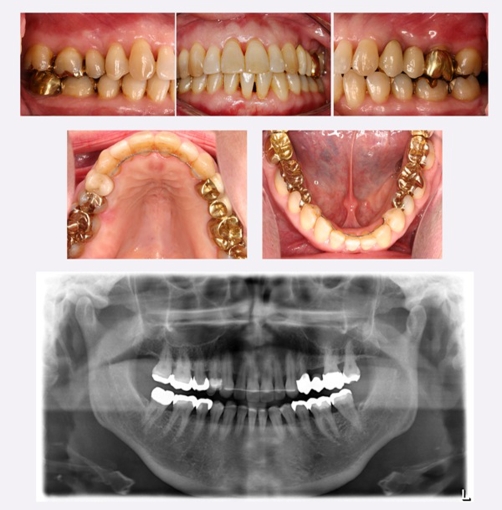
Postactive treatment intraoral photographs and panoramic radiograph.
